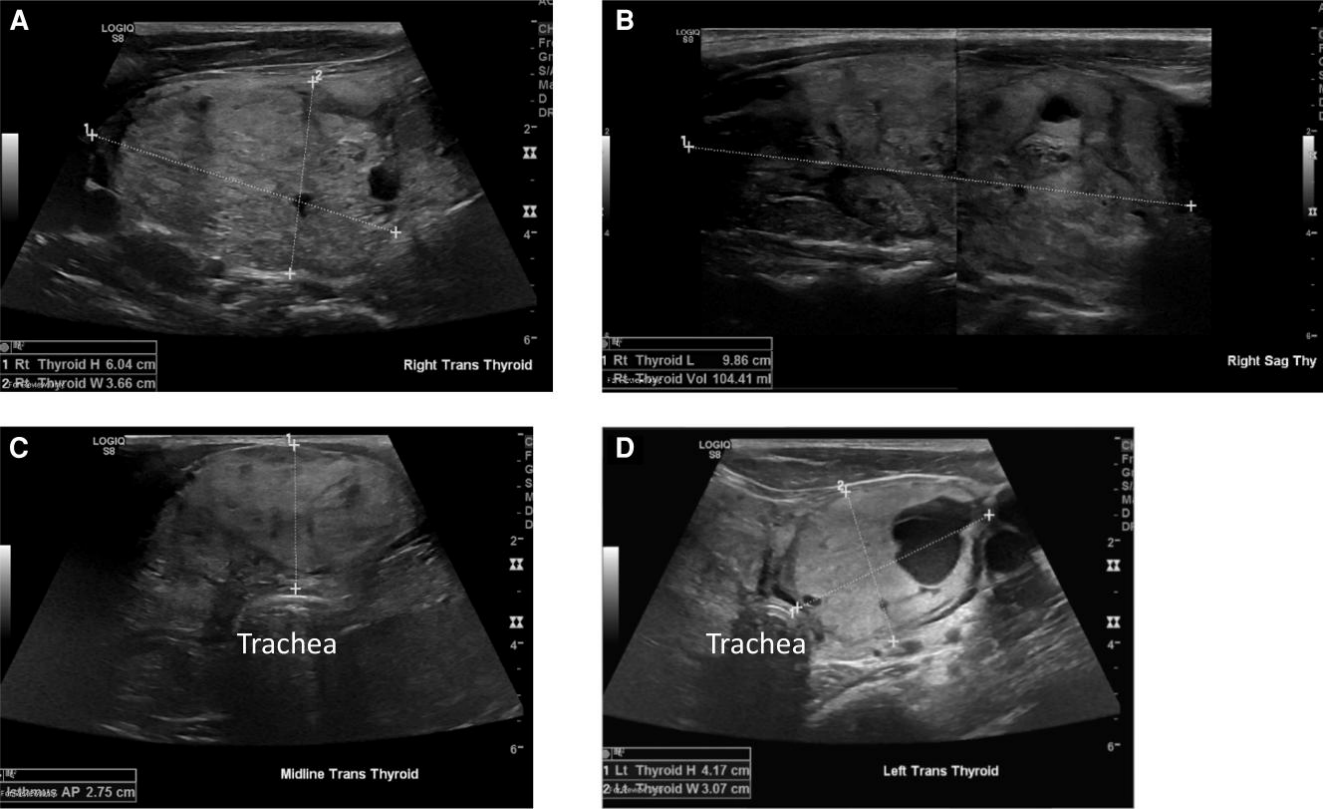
Thyroid ultrasound images 1 month before radiofrequency ablation of right and isthmic nodules. Right nodule in transverse (A) and sagittal (B) views; (C) isthmus nodule; left nodule (D).
